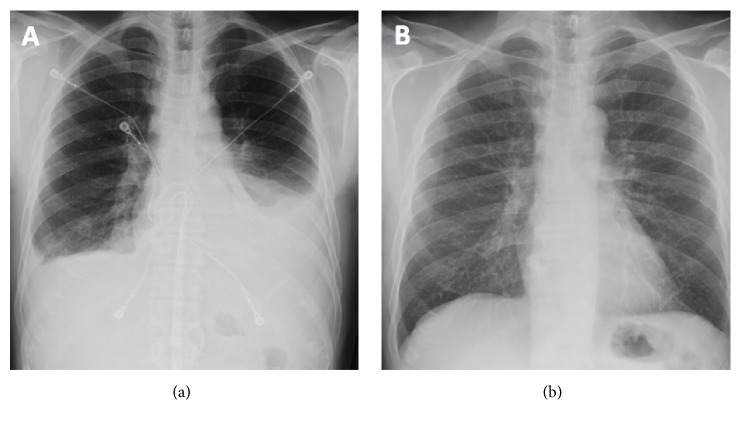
Chest radiograph at admission (a) demonstrates moderate left and trace right pleural effusions with a left retrocardiac opacity. Follow-up chest radiograph shows complete resolution of the abnormalities after treatment (b).
